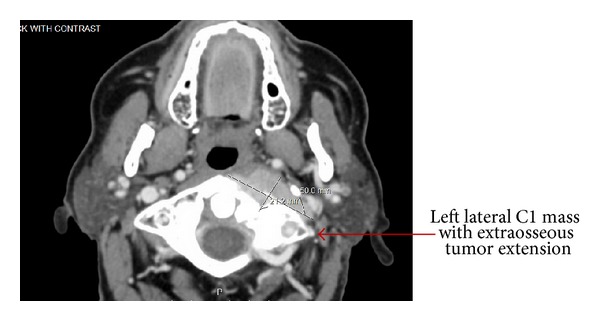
CT scan of left cervical C1 mass.
